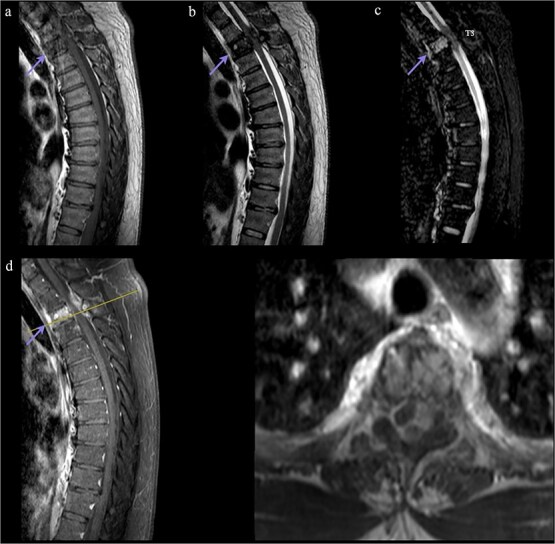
Preoperative MRI. (a, b) T1/T2 sagittal: Hypointense T3 lesion with epidural extension and cord compression. (c) STIR sagittal: Hyperintensity with myelopathic changes (T2–T4). (d) Post-gadolinium sagittal/axial: Contrast enhancement. The line indicates the T3 level. Arrow marks T3 level.
